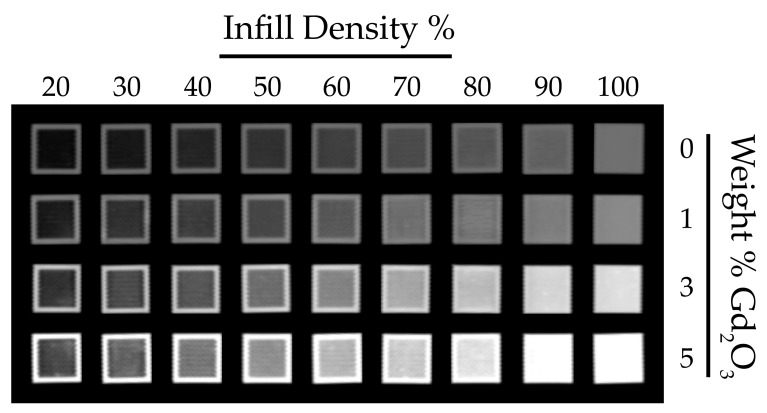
Coronal CT images of PLA/Gd_2_O_3_ calibration cubes with varying infill densities and filler contents.
